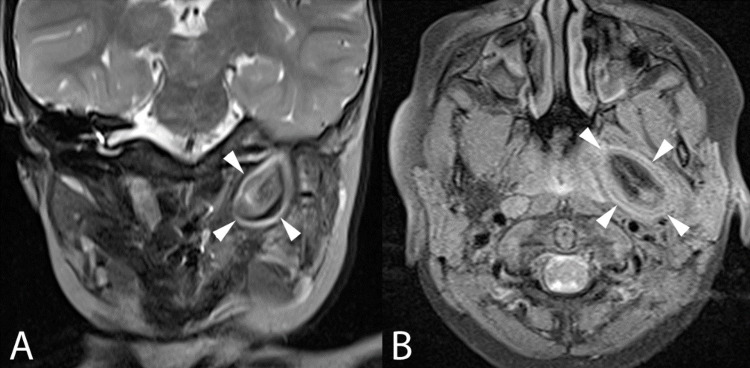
Coronal T2WI MRI (A) of the infratemporal fossa and axial fat-suppressed T1WI MRI (B) of parapharyngeal spaces These sequences depict the signal intensities of the left parapharyngeal lesion. Of note is the signal suppression of the central fatty matrix of the said lesion shown in 4B (white arrowheads), analogous to parapharyngeal and subcutaneous fat. This matrix follows the signal intensity of fat in all sequences. The laminated soft tissue capsule exhibits T2 lengthening (4A, white arrowheads). Musculature within the left masticator and prevertebral spaces are merely displaced and not infiltrated. The lesion abuts the left carotid space without definite encasement MRI: magnetic resonance imaging
